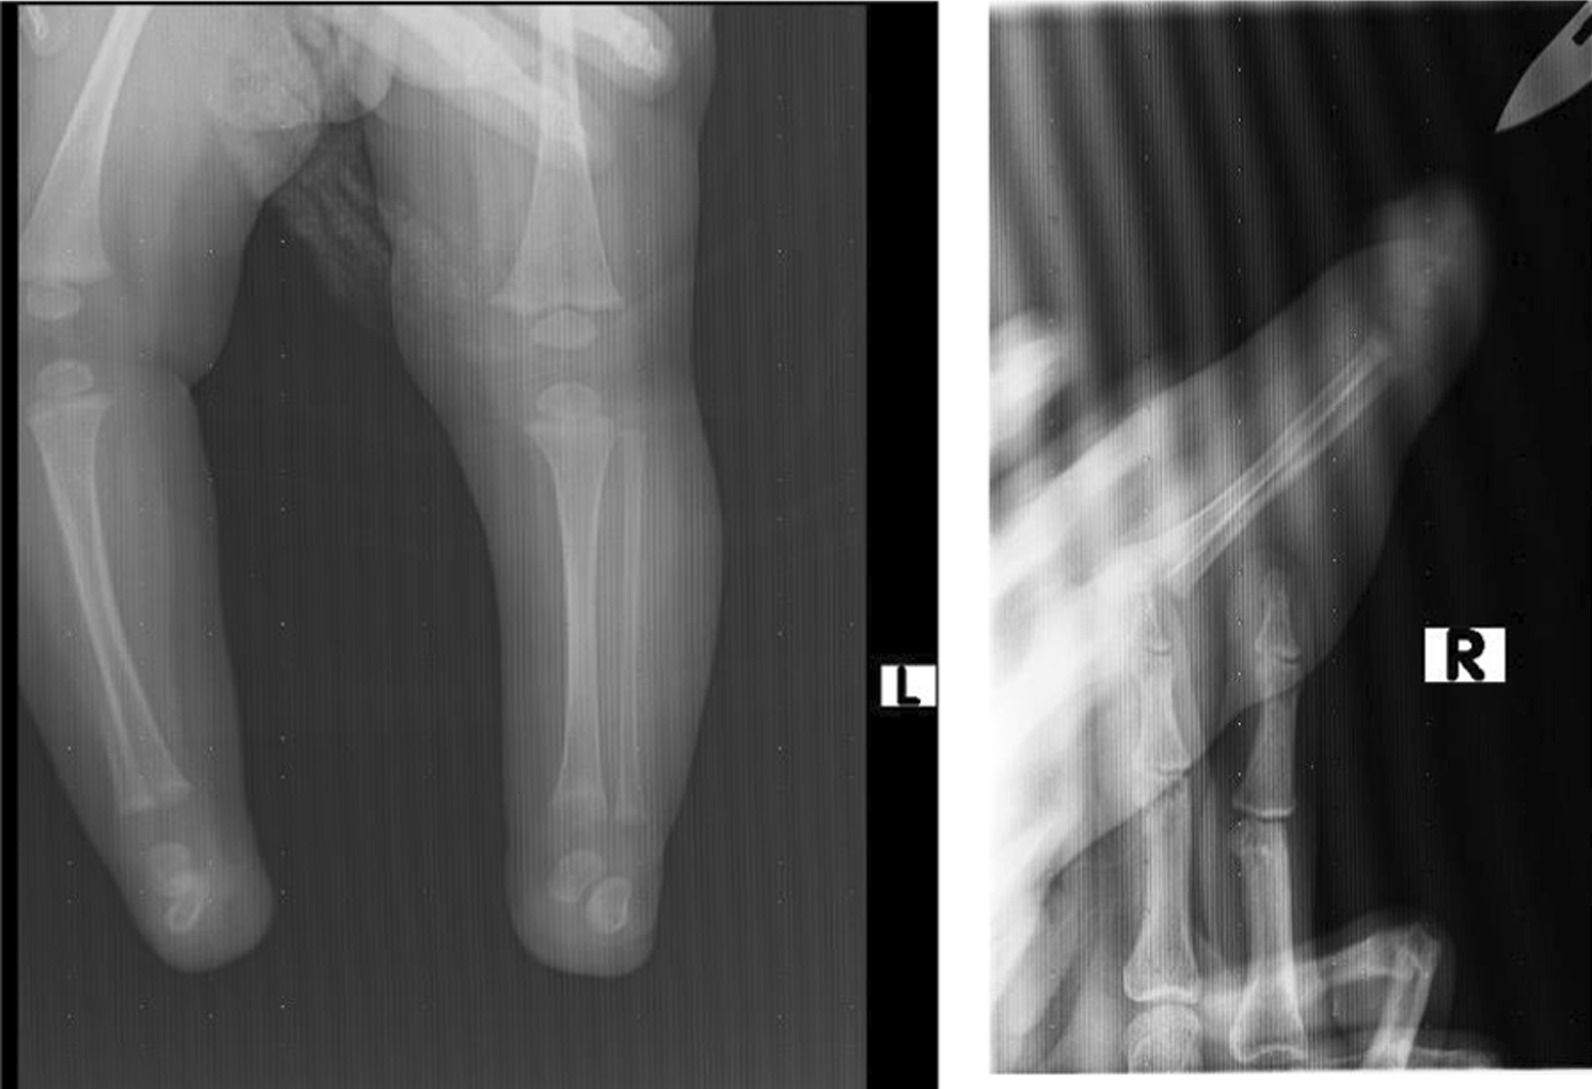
X-ray images of upper (right) and lower (left) limbs for case 2
